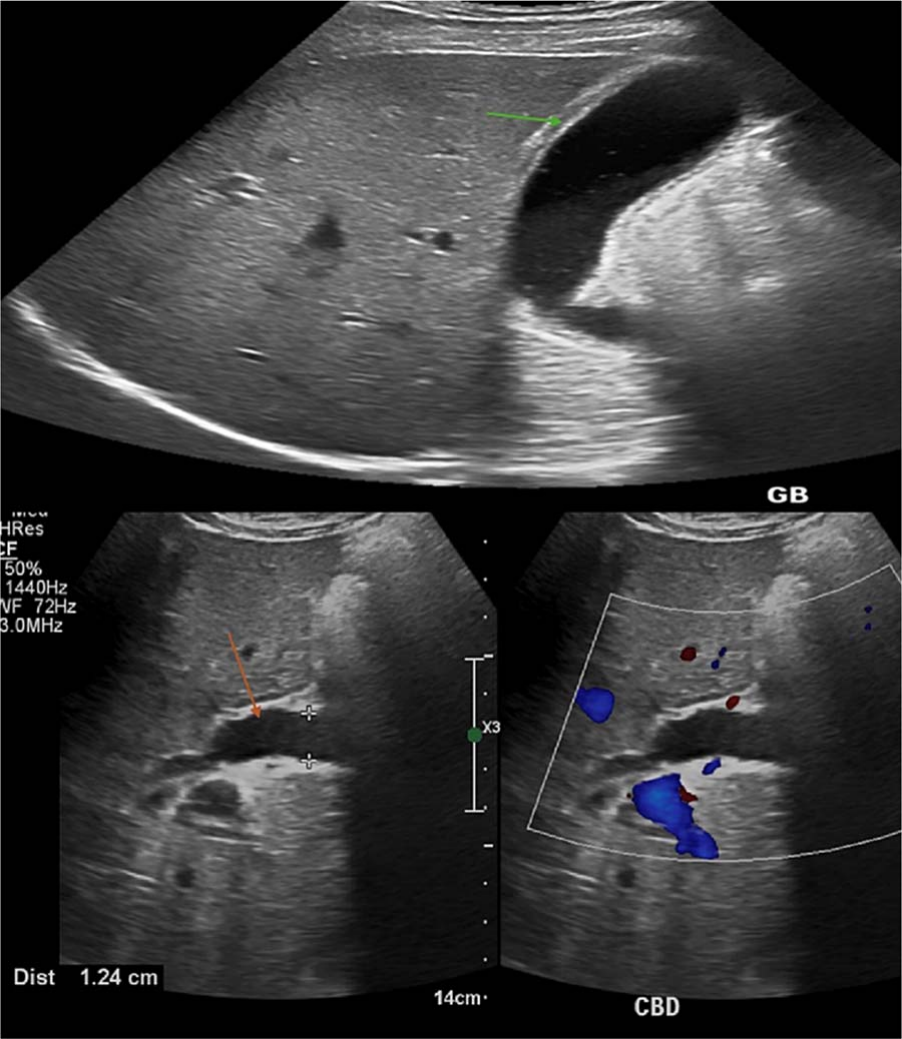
Abdominal axial ultrasound of the liver and gallbladder shows thick edematous gallbladder wall and a dilated common bile duct with intrahepatic biliary dilatation.
